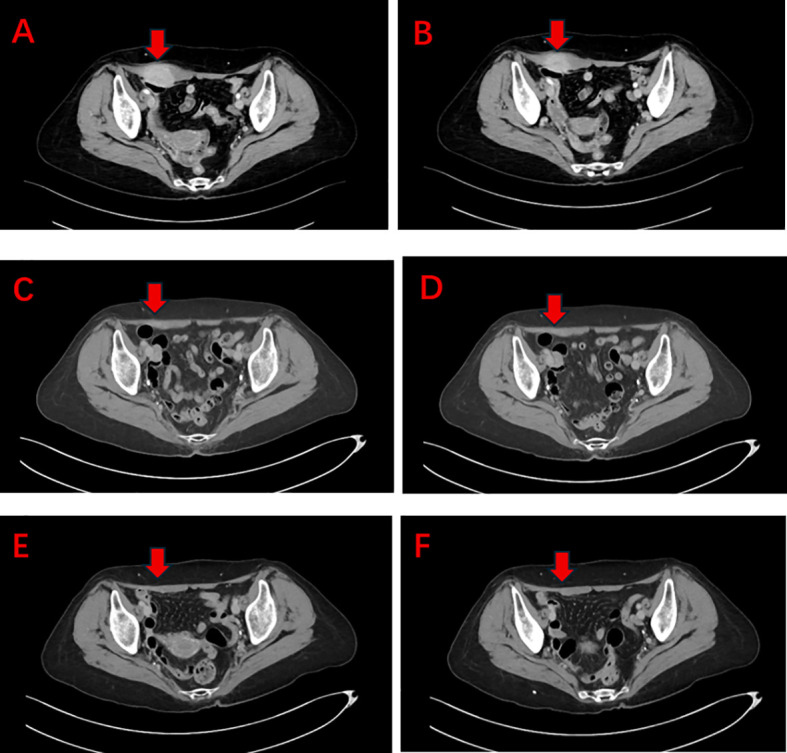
(A, B) CT findings at initial diagnosis. (C, D) Follow-up after 2 cycles of treatment, with therapeutic efficacy evaluated as partial response (PR). (E, F) Follow-up after 4 cycles of treatment, with therapeutic efficacy evaluated as complete response (CR).
